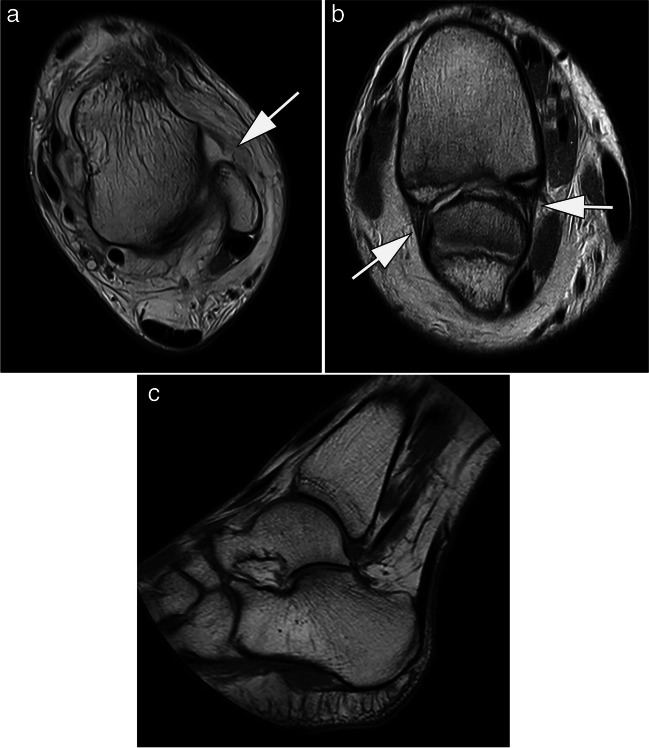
MRI at 0.55 T of the ankle of a 14-year-old patient after ankle sprain. A Axial proton density-weighted image shows a complete rupture of the anterior talofibular ligament (arrow). B Parasagittal proton density-weighted image demonstrates that the anterior and posterior tibiofibular ligaments are intact (arrows). C Small-bore dedicated 1.0-T extremity MRI allows for assessment of the ankle joint, but depending on size of the patient usually plantar flexion is applied as shown in this T1-weighted spin echo image. Note circular field of view of magnet system. TA: 03:07 min (A), 05:26 min (B), 04:21 min (C)
